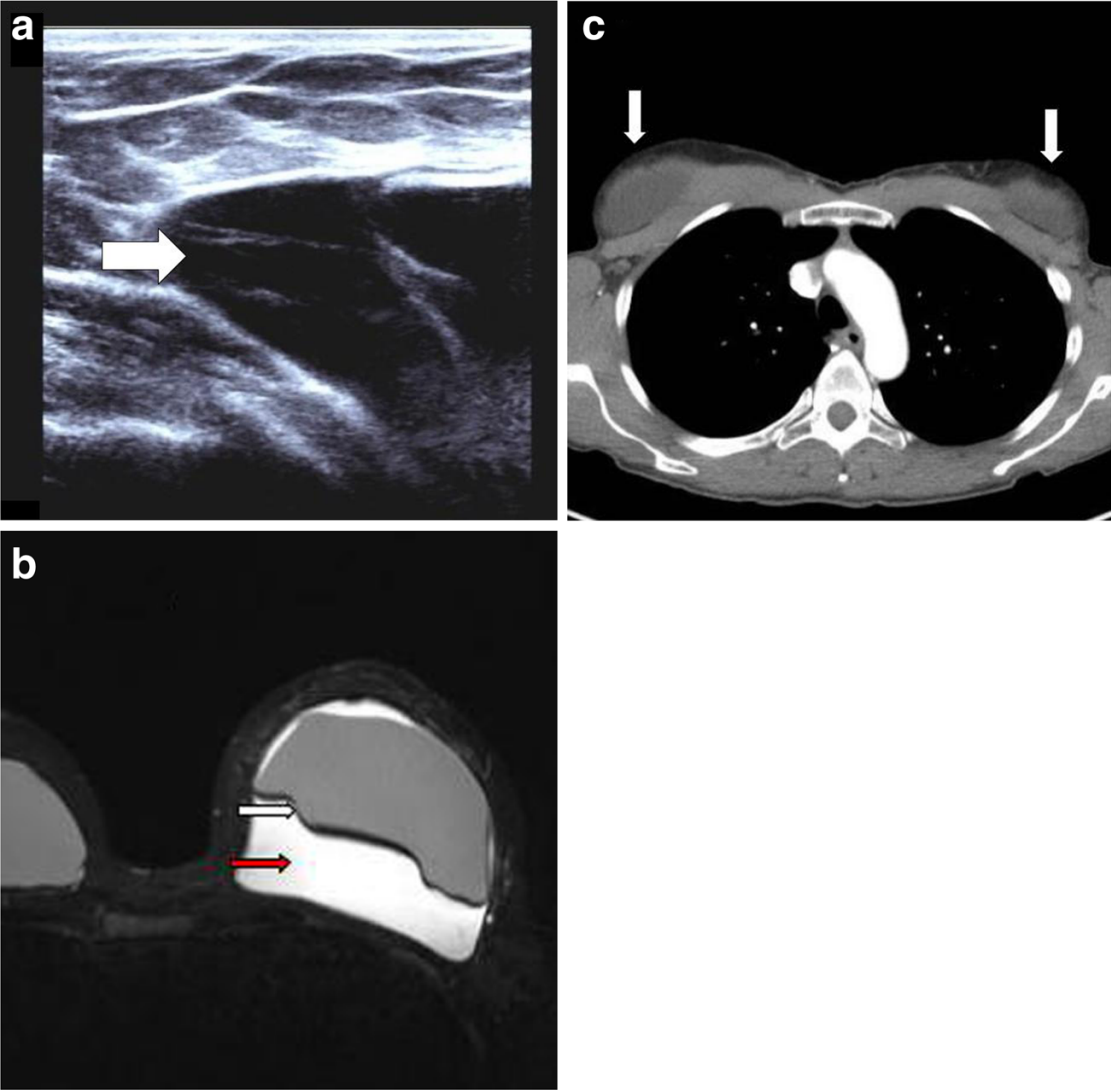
a Ultrasound revealed a large septated seroma (*white arrow*), which was aspirated the following day. Cytology confirmed BIA-ALCL. Ultrasound has a sensitivity of 84% and specificity of 75% for detecting an effusion. These figures are similar or better than CT or magnetic resonance imaging (MRI) in effusion detection [7]. b MRI provides characterisation of the implant’s capsule, defining enhancement and thickening [14, 15]. This makes it the modality of choice for defining the implant capsule (*white arrow*) [7]. BIA-ALCL typically presents as a delayed, non-infective fluid collection surrounding the implant (*red arrow*) or its surrounding scar capsule, with or without evidence of capsular rupture [13]. c Staging CT revealed a small to moderate effusion adjacent to both breast implants (*white arrows*)
